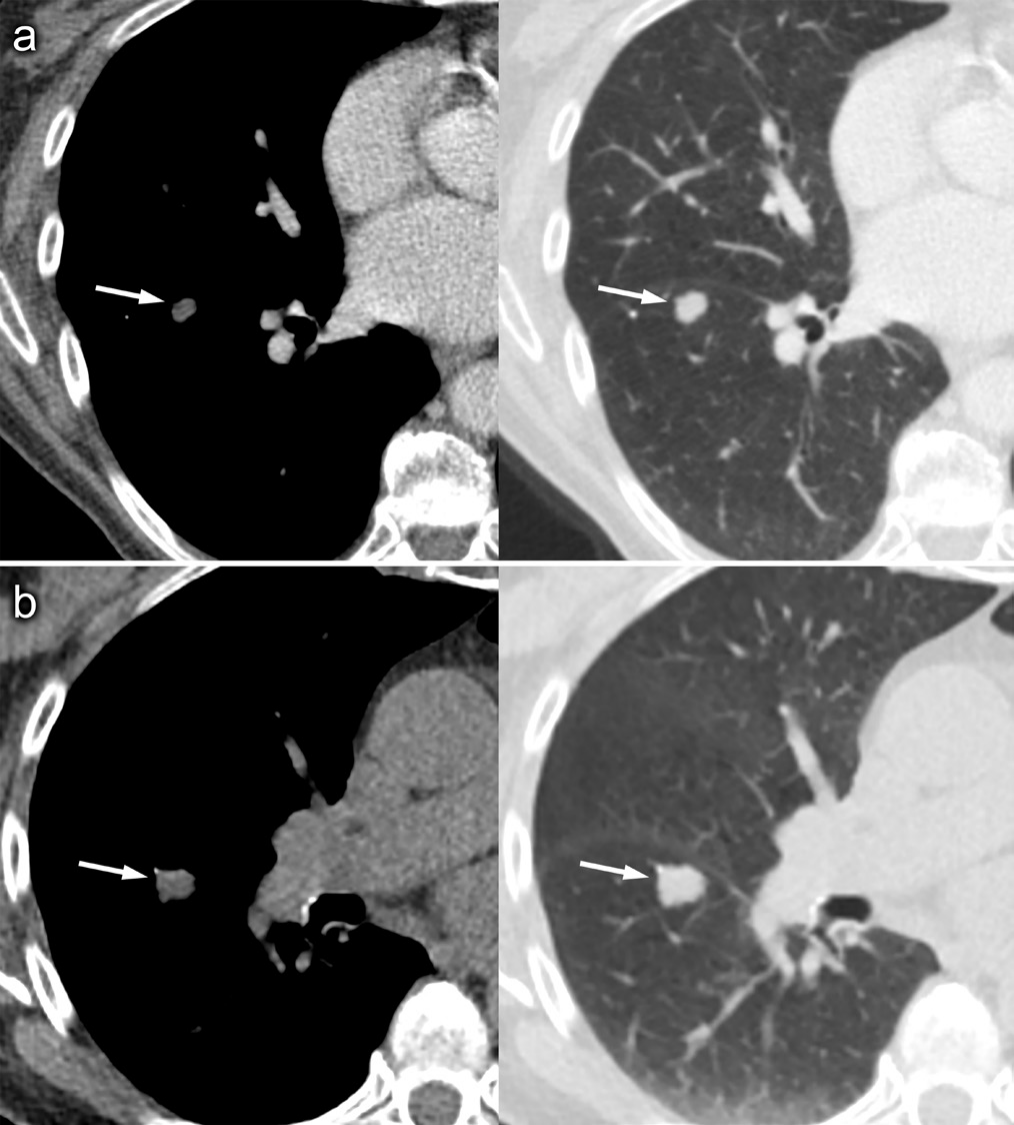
Axial CT images on soft-tissue and lung window settings on post-contrast CT (A) and the CT component of a non-contrast PET-CT (B) performed 6 weeks later, which show an enlarging low-density pulmonary nodule within the lower lobe of the right lung. Note that image A suggests the presence of macroscopic fat anteriorly within the lesion. PET, positron emission tomography.
